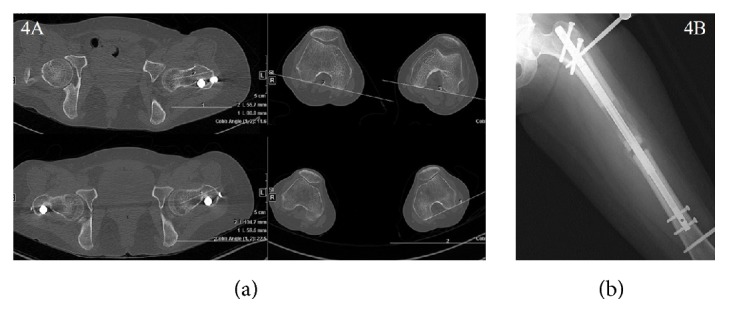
(a) A 38-year-old woman who underwent bilateral femur lengthening complained that she abruptly cannot be able to control her left leg, just after “popping sense” on the leg during early consolidation phase. To confirm the failure of rotational stability of the nail, the CT scans of lower extremity with maximal internal and maximal external rotation were taken, respectively. The femoral rotational alignment changed to about 20 degrees according to the patient's position. (b) Additional mono-fixator was applied to gain a proper rotational alignment and stability until the consolidation is finished.
